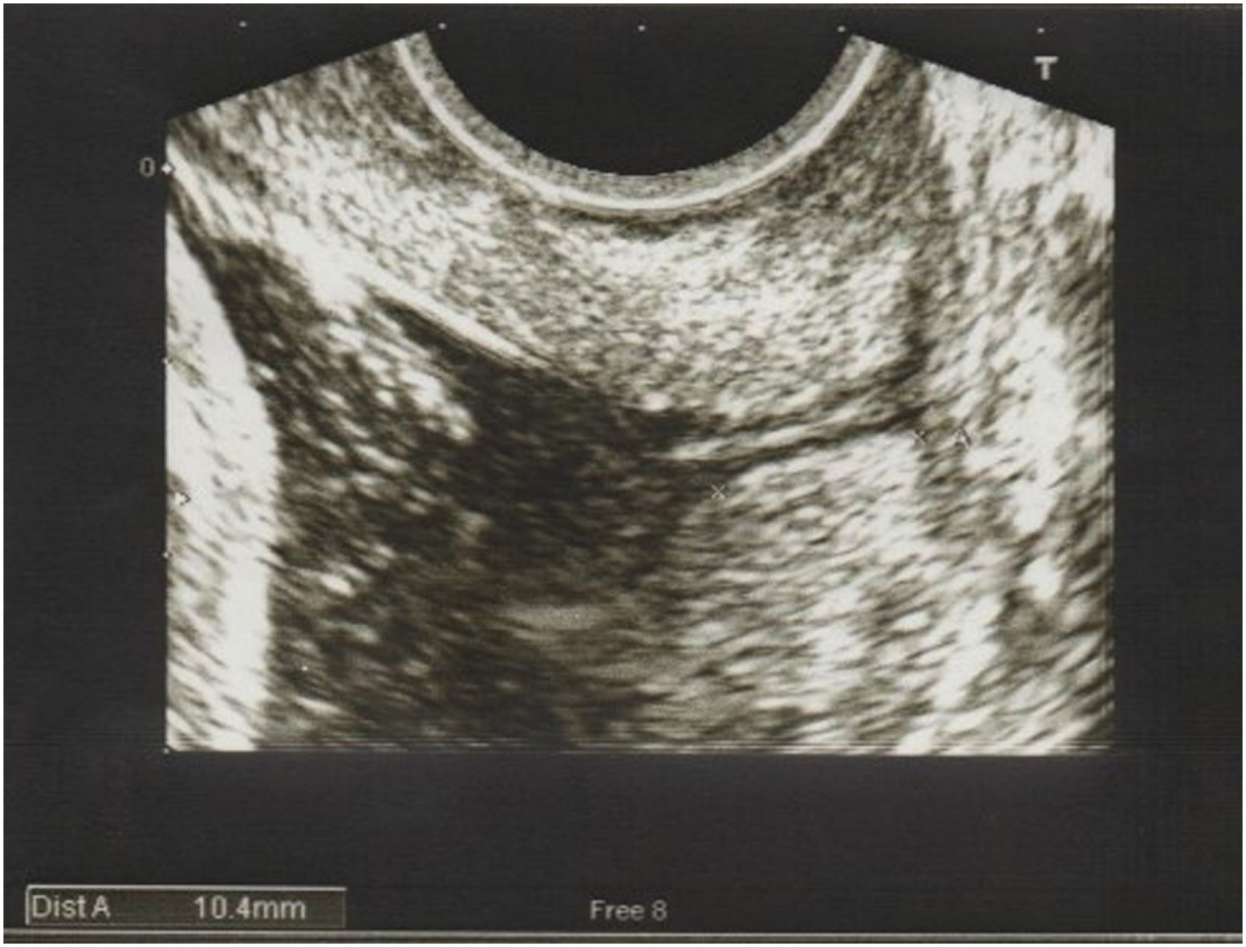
Transvaginal ultrasound scan of cervix, showing length of 10.4 mm.
